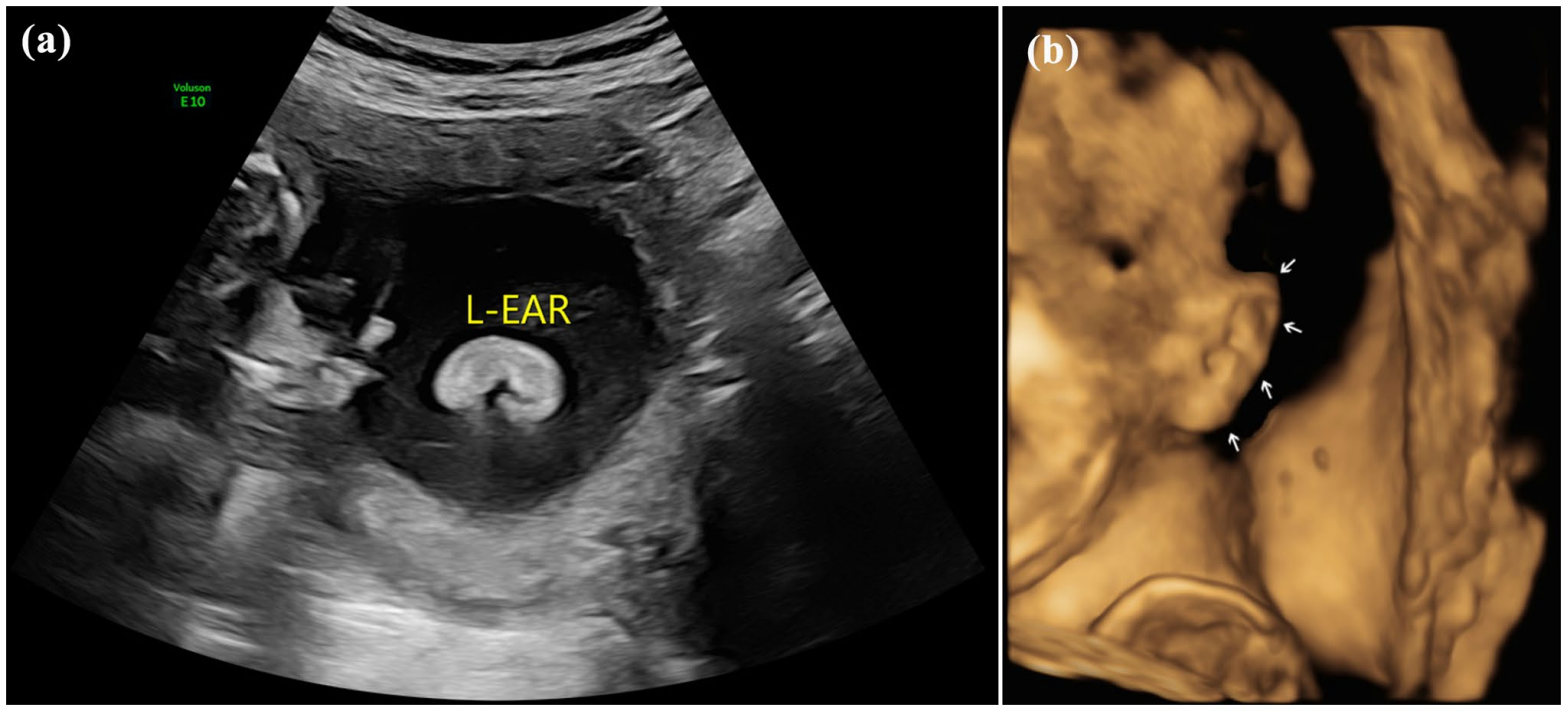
Images of normal fetal ear: (A) 2D sonography and (B) 3D sonography.
